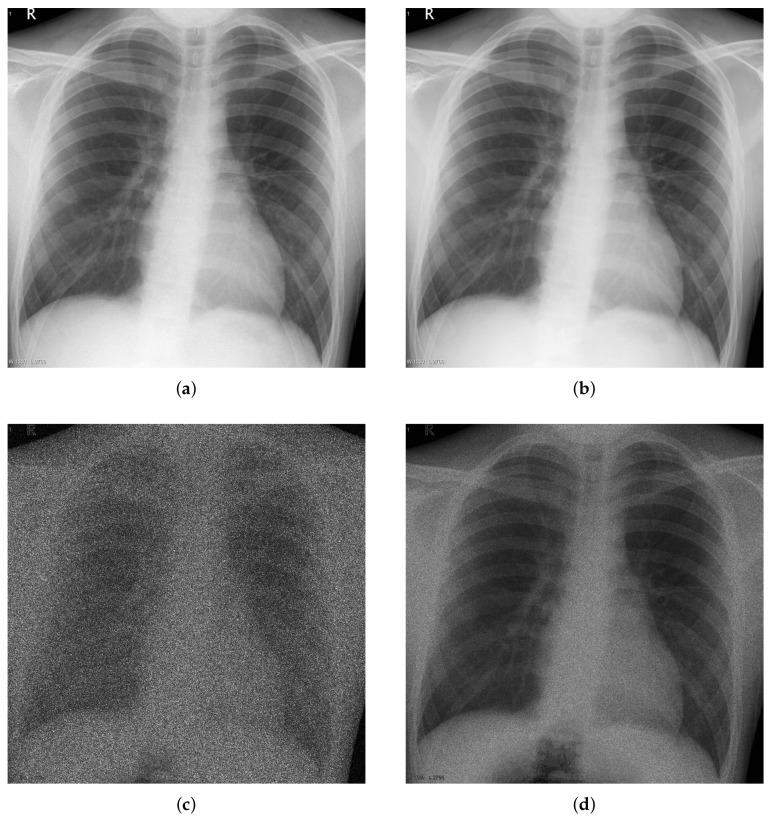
Noise analysis of Poisson noise. (a) Poisson noise-added image; (b) noiseless image (high-dose X-ray image); (c) noise image (artificially generated); (d) variance of noise images.
